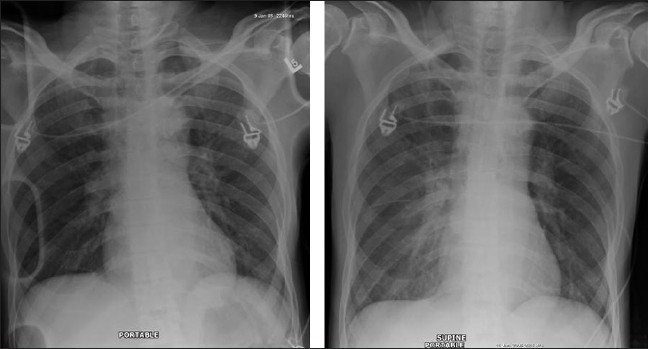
Figures 8, 9 and 10 show a series of chest x-rays and CT scans over a period of 18 hours of a patient following blunt thoracic trauma. The initial chest x-ray [Figure 8] appears normal
